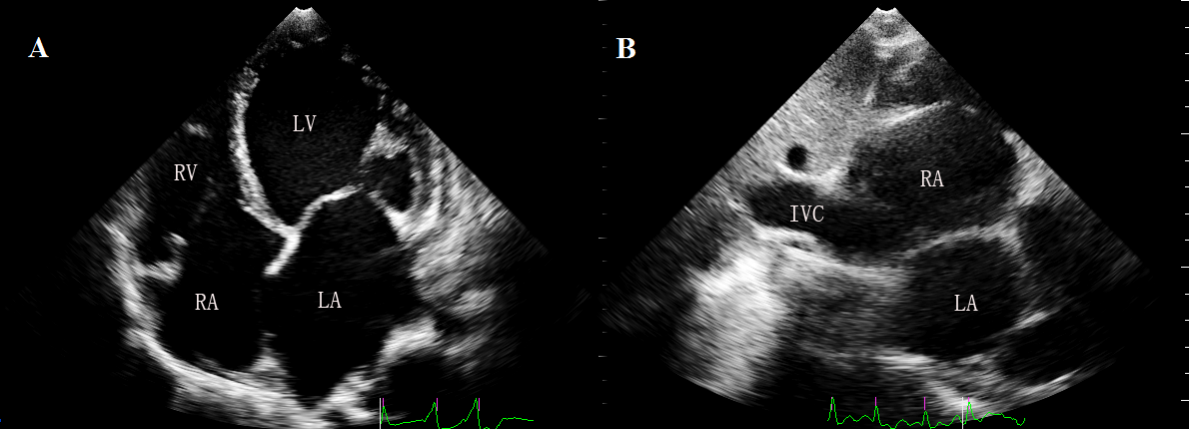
Follow up after 1 week anticoagulation: In A) apical 4-chamber view and B) subxyphoid view shows complete disappearance of the thrombi. LV = left ventricle; RA = right atrium; RV = right ventricle, LA = left atrium, IVC = inferior vena cava.
